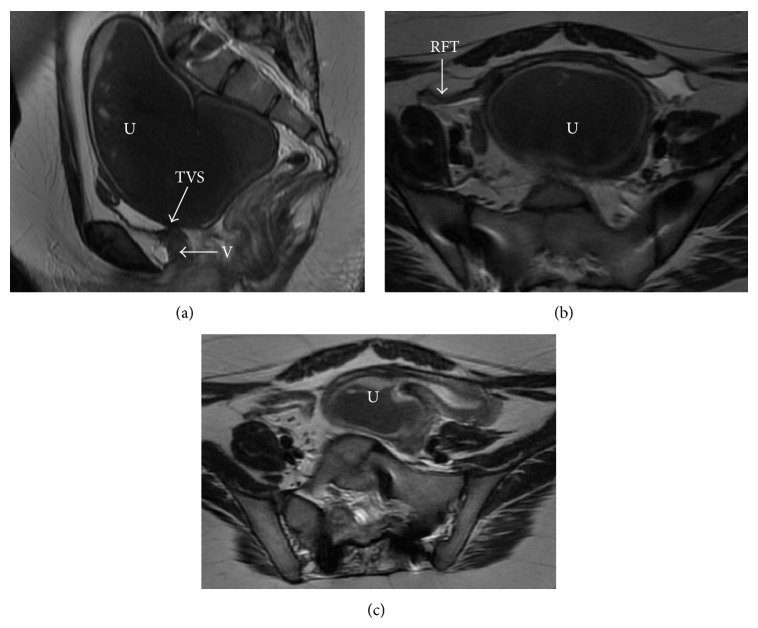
MRI of the pelvis, demonstrating site of transverse vaginal septum. (a) Sagittal T2 image, showing uterus distended with blood (U), the site of transverse vaginal septum (TVS), and narrowing at the level of vagina (V). (b) Axial T2 image, demonstrating distended uterus (U). (c) Axial T2 image, showing the distended left unicornuate uterus. U: uterus, TVS: transverse vaginal septum, V: vagina, and RFT: right fallopian tube.
